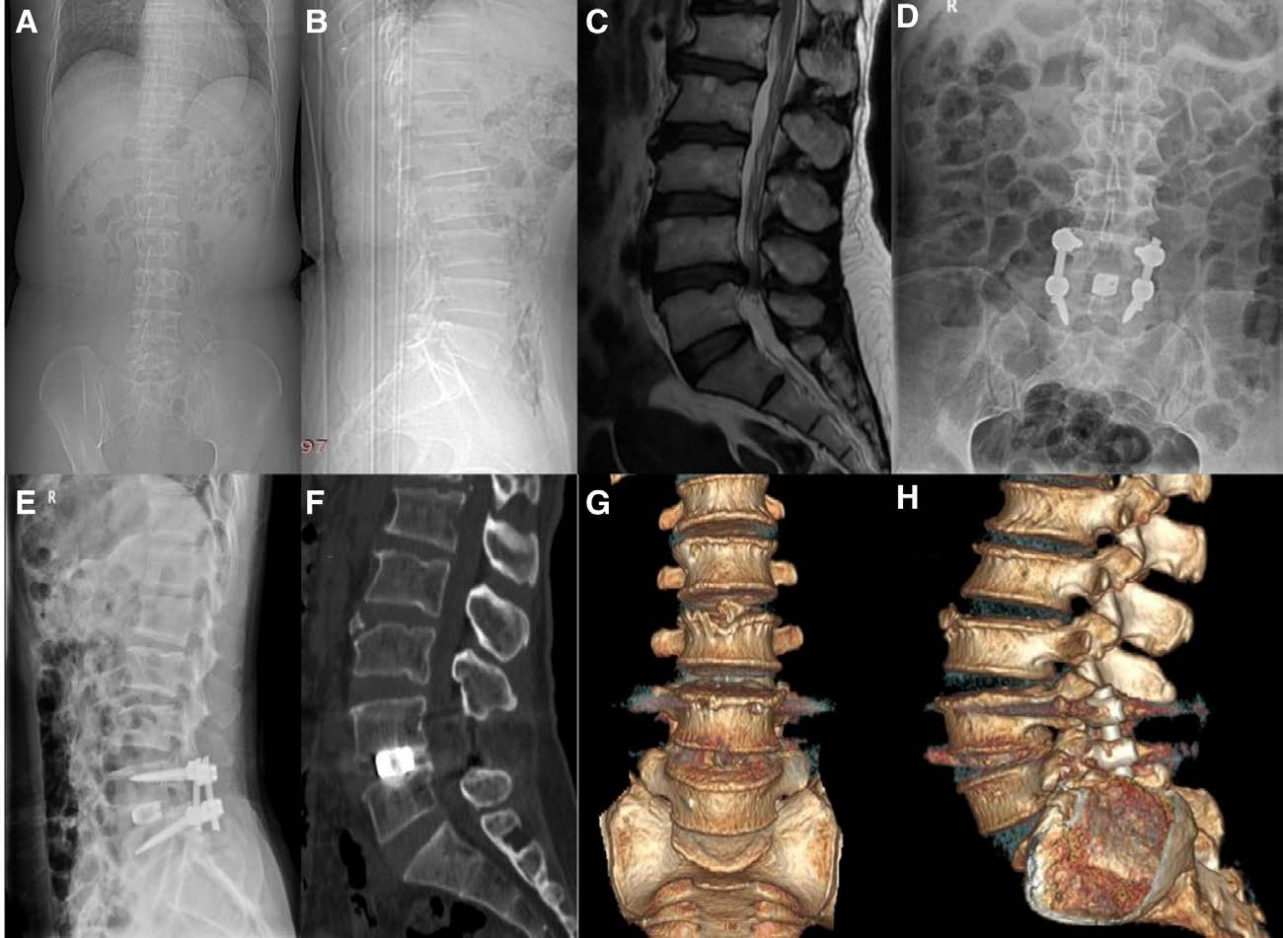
A 51-year-old female patient diagnosed as lumbar spinal stenosis. We evaluated her preoperative clinical outcome. The JOA score was 13, the ODI index was 66%, the PFs was 1, the VAS was 6 and the SF-36 score was 42.5%. And here were her radiographic measurements before surgery. The intervertebral disc height was 9.45 mm, the foraminal height was 16.5 mm, the foraminal area was 108.7 mm², the segmental lumbar lordosis angle was 18.7°, the lumbar lordosis angle was 36.5°. We measured the radiographic data immediately after the PLIF surgery on L4/L5. The intervertebral disc height was 11.8 mm, the foraminal height was 17.9 mm, the foraminal area was 115.4 mm², the segmental lumbar lordosis angle was 20.5°, the lumbar lordosis angle was 42.4°. We evaluated her clinical outcome at 6-month postoperative. The JOA score was 27, the ODI index was 10%, the PFs was 4, the VAS was 0 and the SF-36 score was 92.5%. (A and B) Anterior and lateral X-ray of lumbar spine before surgery. (C) Lumbar MRI before surgery. (D and E) 6-month postoperative anterior and lateral X-ray of lumbar spine. (F–H) 6-month postoperative lumbar CT and three-dimensional reconstruction. JOA = The Japanese Orthopaedic Association Evaluation treatment score, ODI = Oswestry Disability Index, PFs = prolo function score, PLIF = posterior lumbar interbody fusion, VAS = pain visual analogue score.
